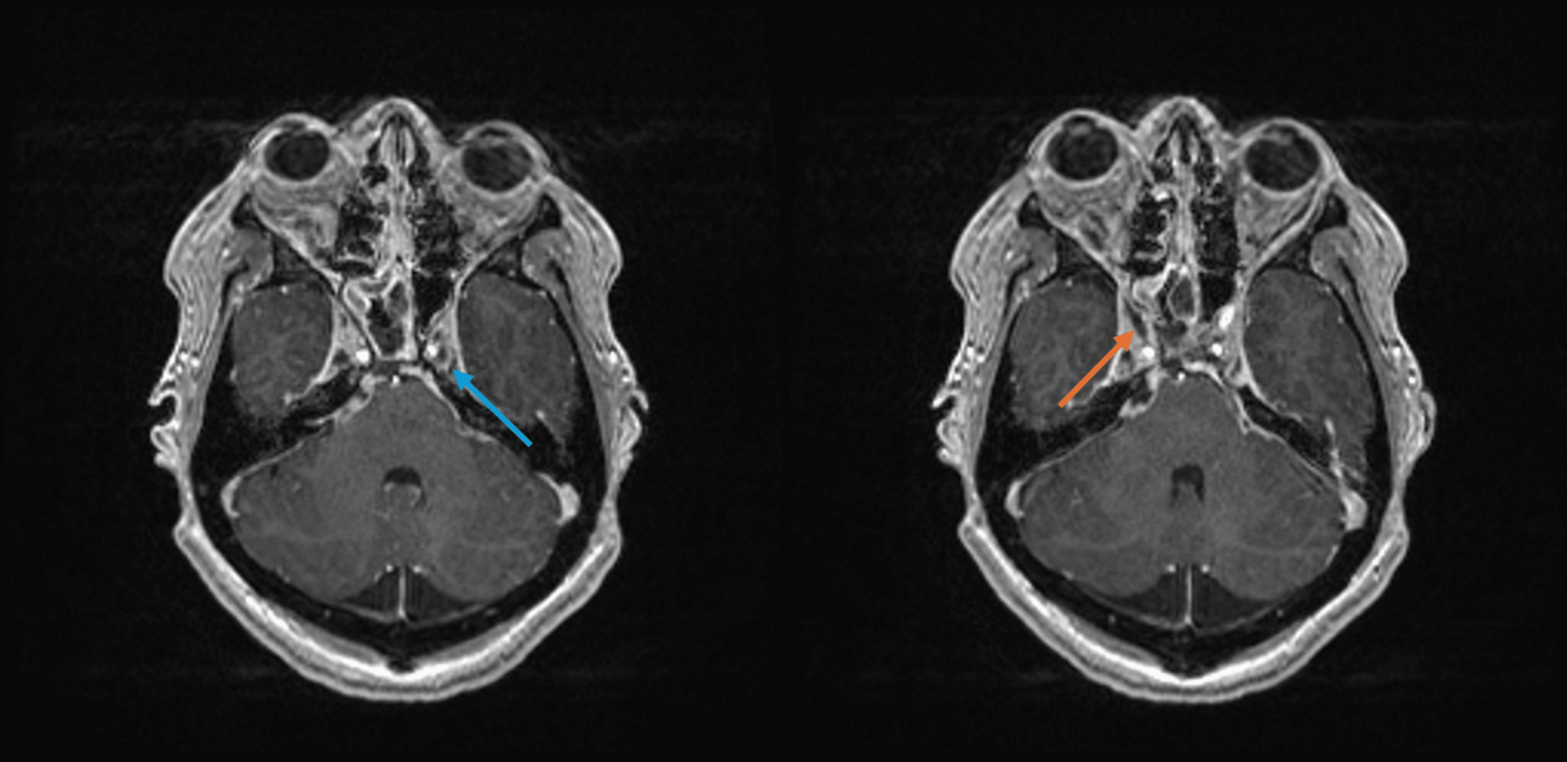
MRI brain: T1-weighted. The blue arrow points to an area with a lack of enhancement within the left cavernous sinus, while the orange arrow points to small filling defects within the right cavernous sinus, measuring approximately 1.3 cm x 0.4 cm. There is diffuse heterogeneous enhancement throughout the cavernous sinuses with asymmetric thickening on the right compared to the left.
